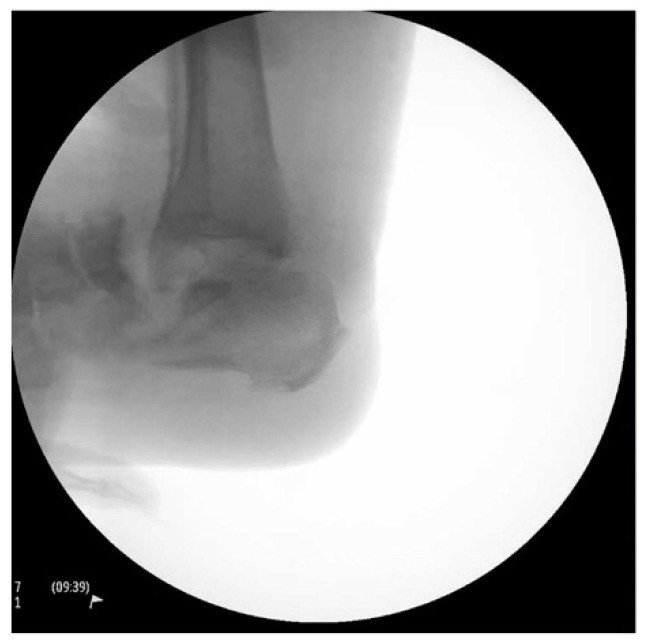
Intraoperative radiograph.
